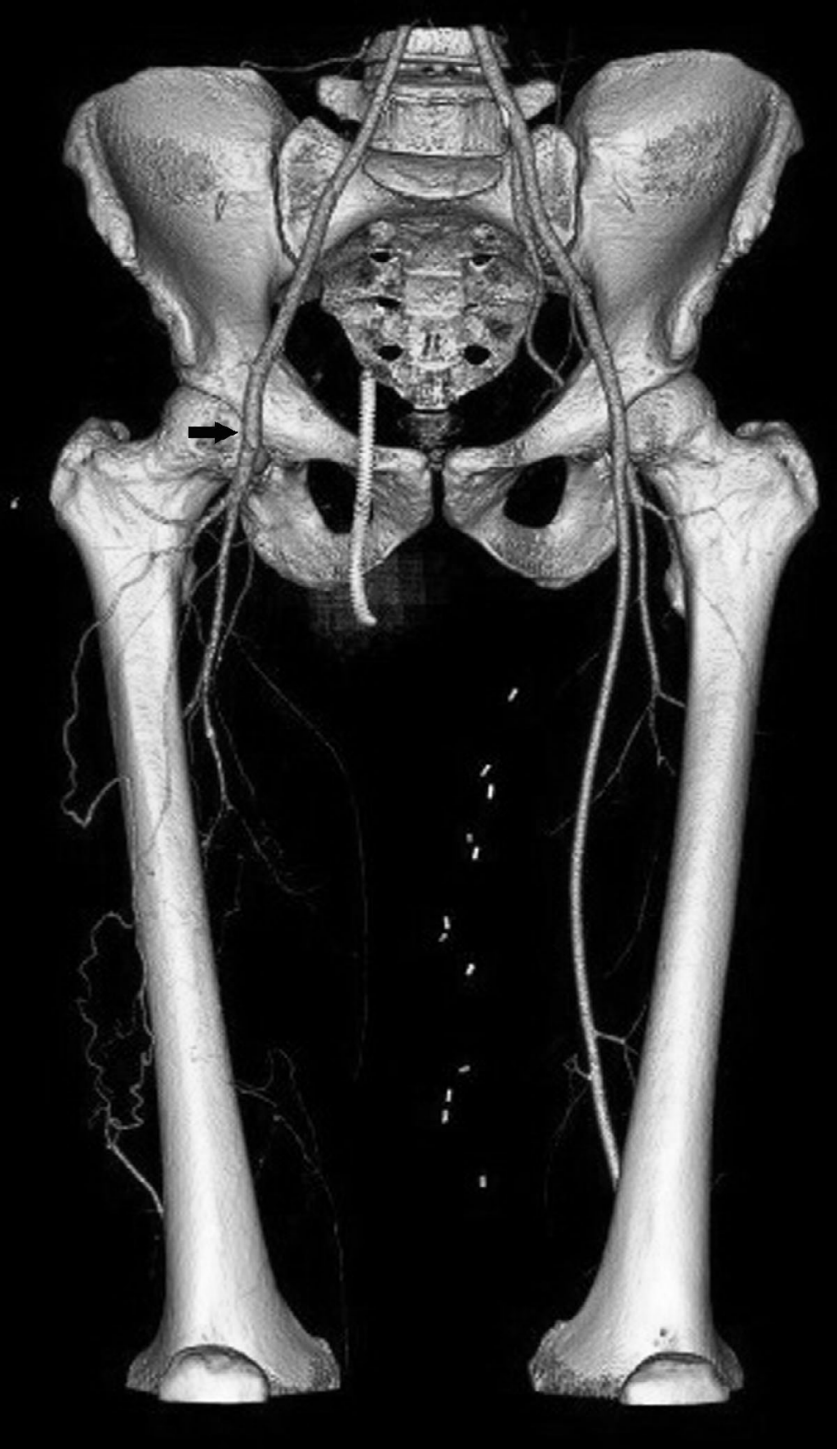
Computed tomography angiography. 3D reconstruction image demonstrates a
